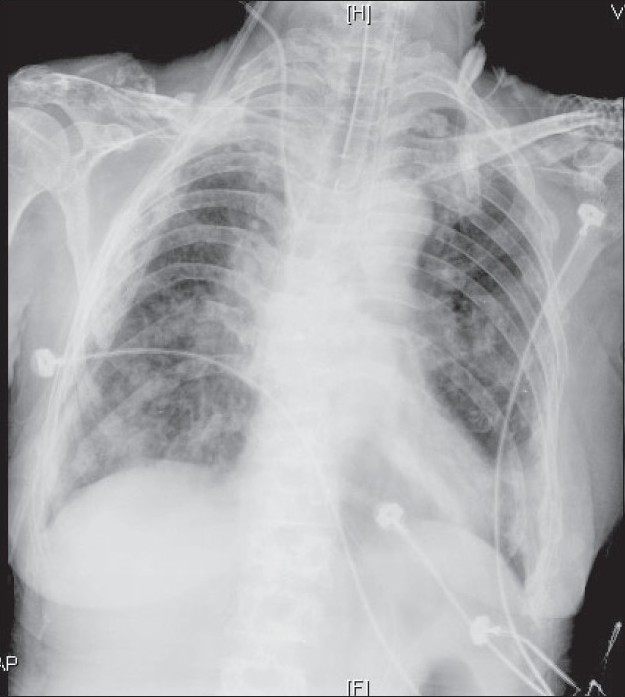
Chest radiograph immediately after intubation
